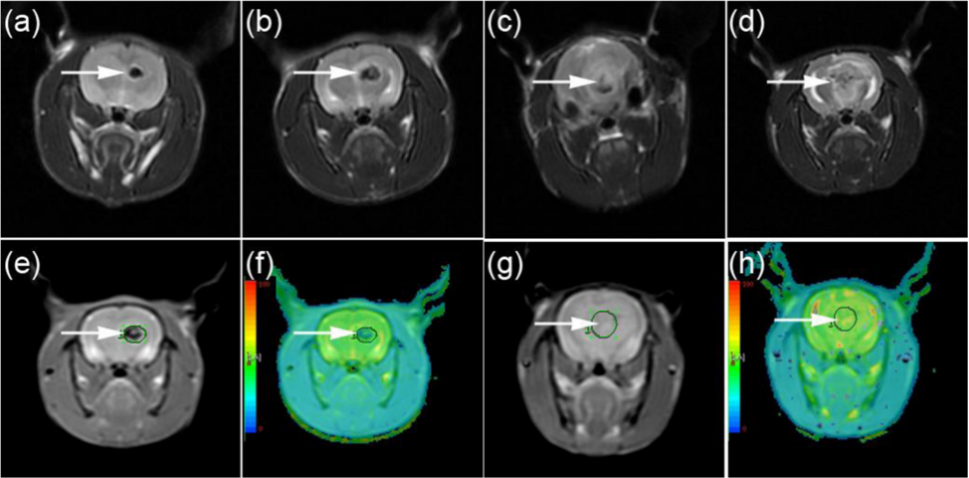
MR imaging of C6 glioma xenograft tumor model. T2-weighted MR images of C6 glioma xenografts that were labeled with 25 μg/mL acetylated APTS-coated Fe_3_O_4_ NPs at (a) 7 days, (b) 14 days, (c) 21 days, and (d) 28 days. (e) The *R*_2_ mapping of C6 glioma xenografts that were labeled with 25 μg/mL acetylated APTS-coated Fe_3_O_4_ NPs at 14 days. (f) A pseudocolor picture of (e). (g) The *R*_2_ mapping of C6 glioma xenografts without labeling at 14 days as a control. (h) A pseudocolor photo of (g). The white arrows indicate the glioma xenografts.
